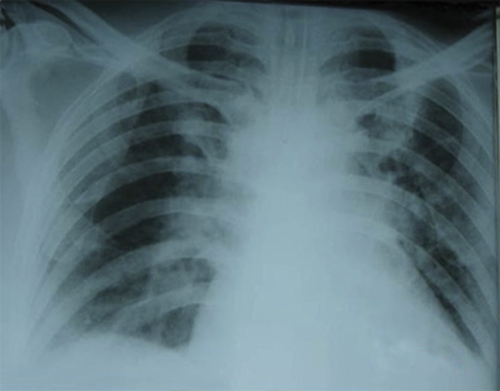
Chest x-ray showing bilateral alveolar infiltrates, predominantly in the right lung and bilateral pleural effusion. Note the marked widening of the mediastinum.
